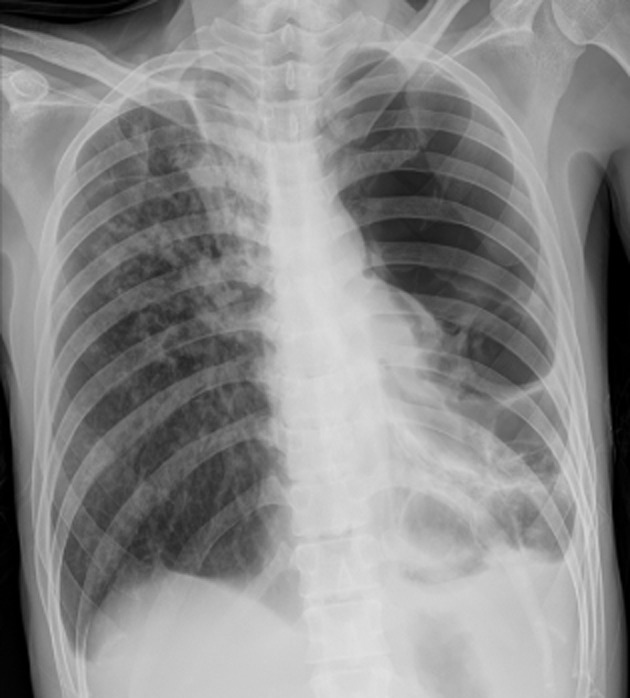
Chest radiograph showing massive pulmonary cavities in the left lung. Extensive areas of lucency in the left lung were present, resulting in the misdiagnosis of pneumothorax and unnecessary chest tube insertion. Patchy opacities and nodularities can be visualized on the right lung field.
